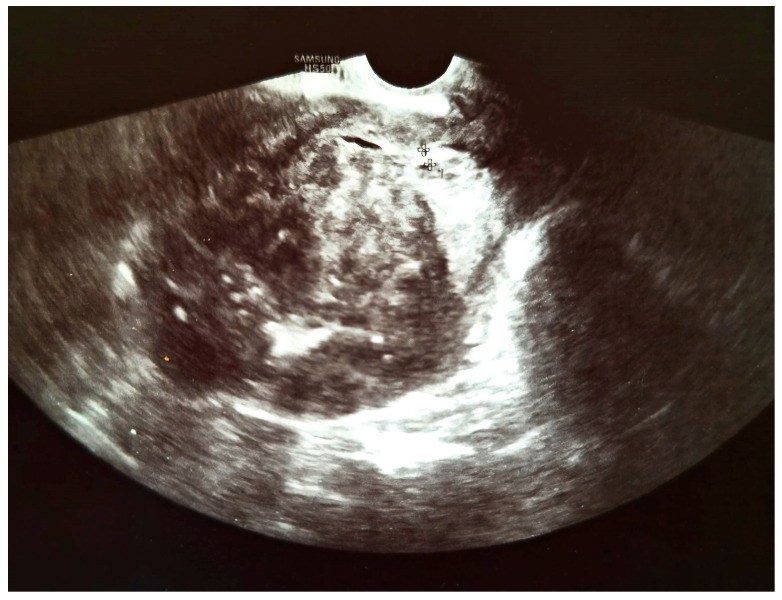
Transvaginal ultrasound image (longitudinal plane) demonstrating an endometrial thickness of 3.4 mm.
